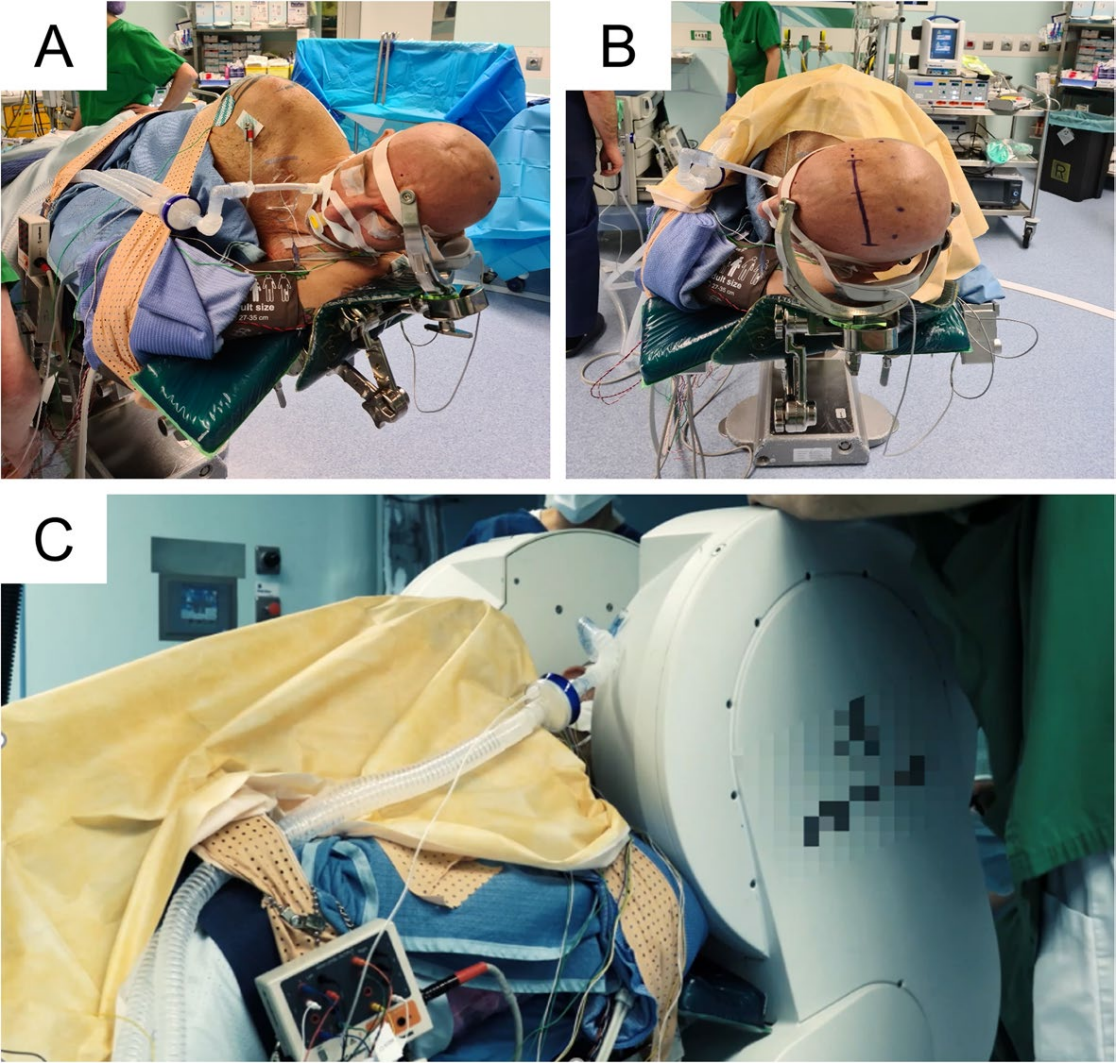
Patient positioning for contralateral transfalcine approach from left to right (A) and low-field MRI setup for intraoperative scanning (B)
